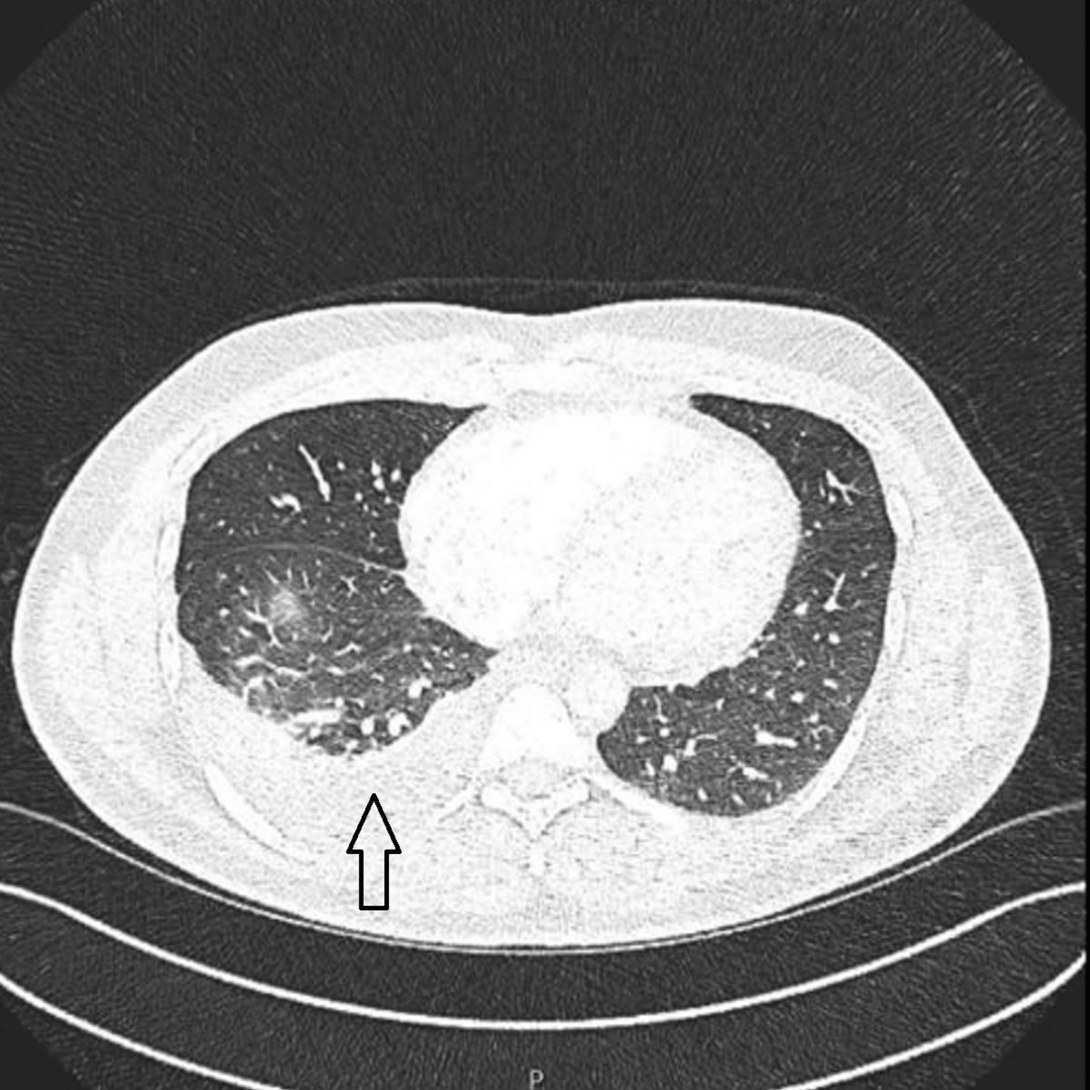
CT pulmonary angiography showing no pulmonary embolism but showing small right-sided pleural effusion and right lower lobe consolidations.
